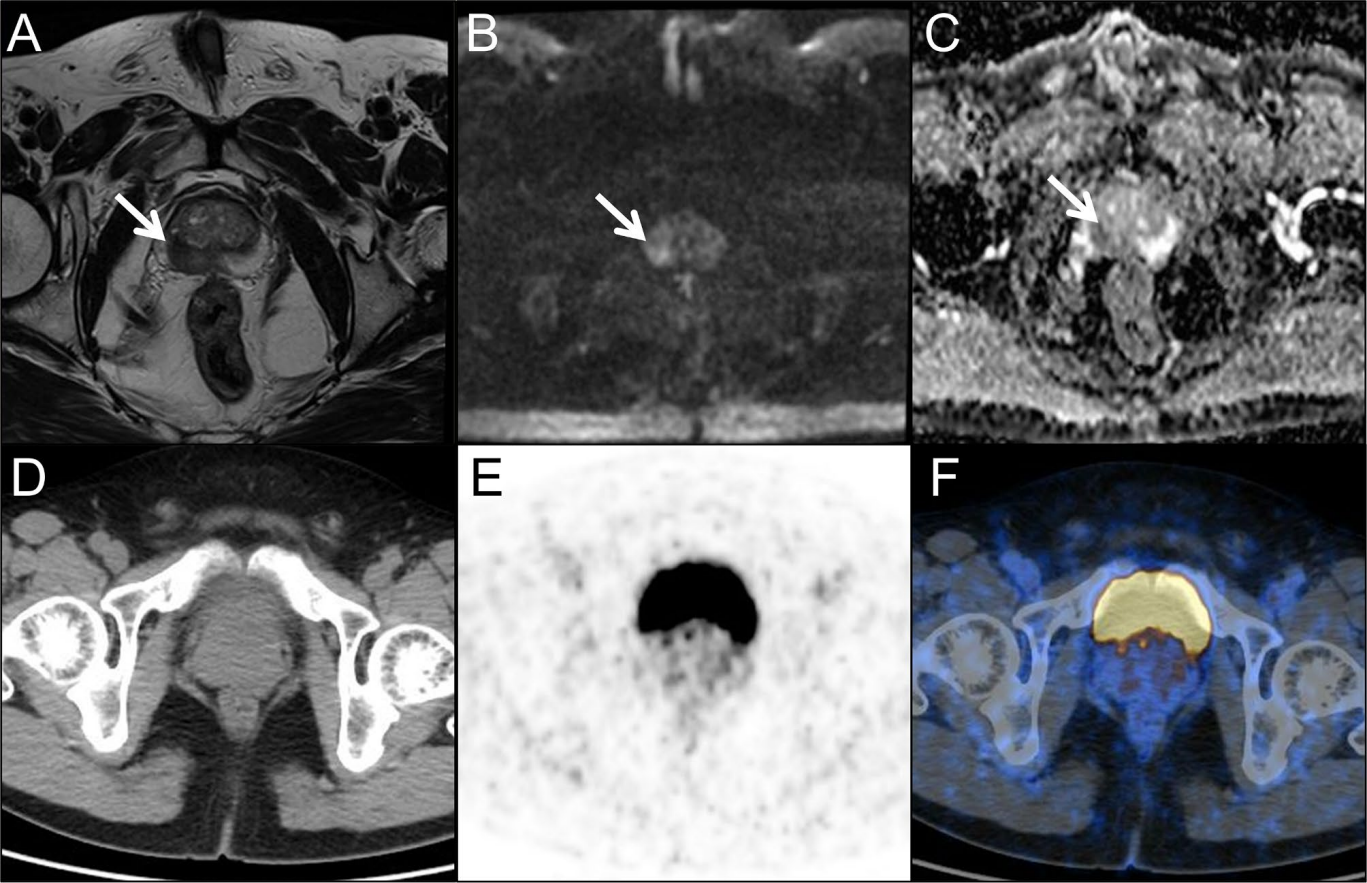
A 66-year-old man presented with a persistently elevated prostate-specific antigen (PSA) range of 7.08–8.58 ng/ml over a period of six months. T2-weighted imaging (A) revealed a hypointense lesion in the right periphery of the prostate (arrow) showing hyperintense on the diffusion weighted imaging (DWI) (B, arrow), and hypointense on the apparent diffusion coefficient (ADC) maps (C, arrow) resulting in a Prostate Imaging-Reporting and Data System (PI-RADS) score of 4. However, positron emission tomography/computed tomography (PET/CT) images (D, CT; E, PET; F, fusion) showed no prostate-specific membrane antigen (PSMA) uptake in the involved region. According to the predictive model, the probability of clinically significant prostate cancer for this lesion is 0.10 (< 0.35). Subsequent prostate biopsy result in benign prostatic hyperplasia accompanied by prostatitis. Following 1-year of symptomatic treatment, the patient’s PSA returned to normal
